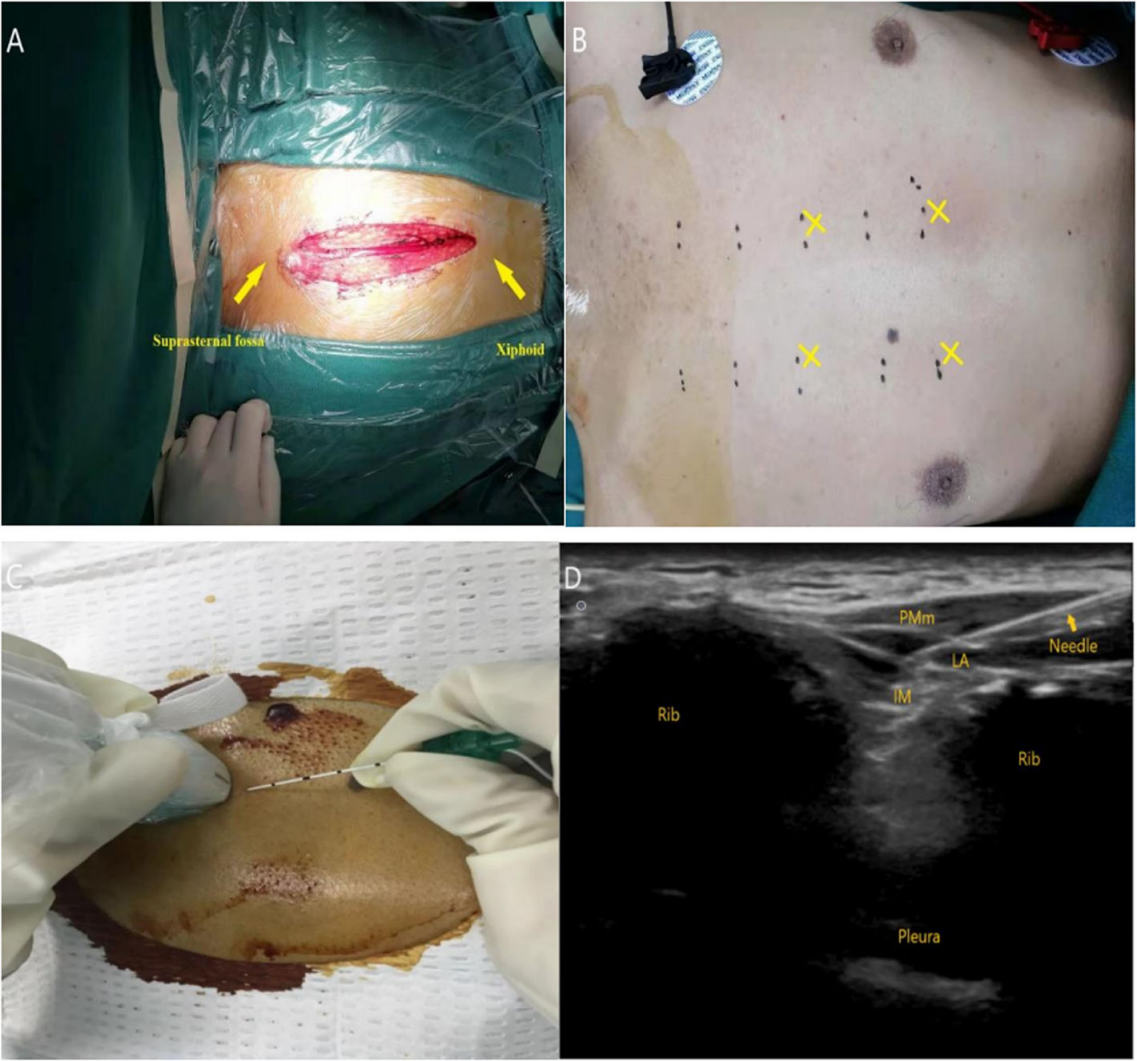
a Surgical incision of patients with median sternotomy for mediastinal mass resection. b Anatomic localization and puncture points. c Position of the ultrasound transducer and needle. d Ultrasound image of the puncture rout and the spread of solution for PSI block. PMm, pectoralis major muscle; IM, intercostal muscle; LA, local anesthetic
